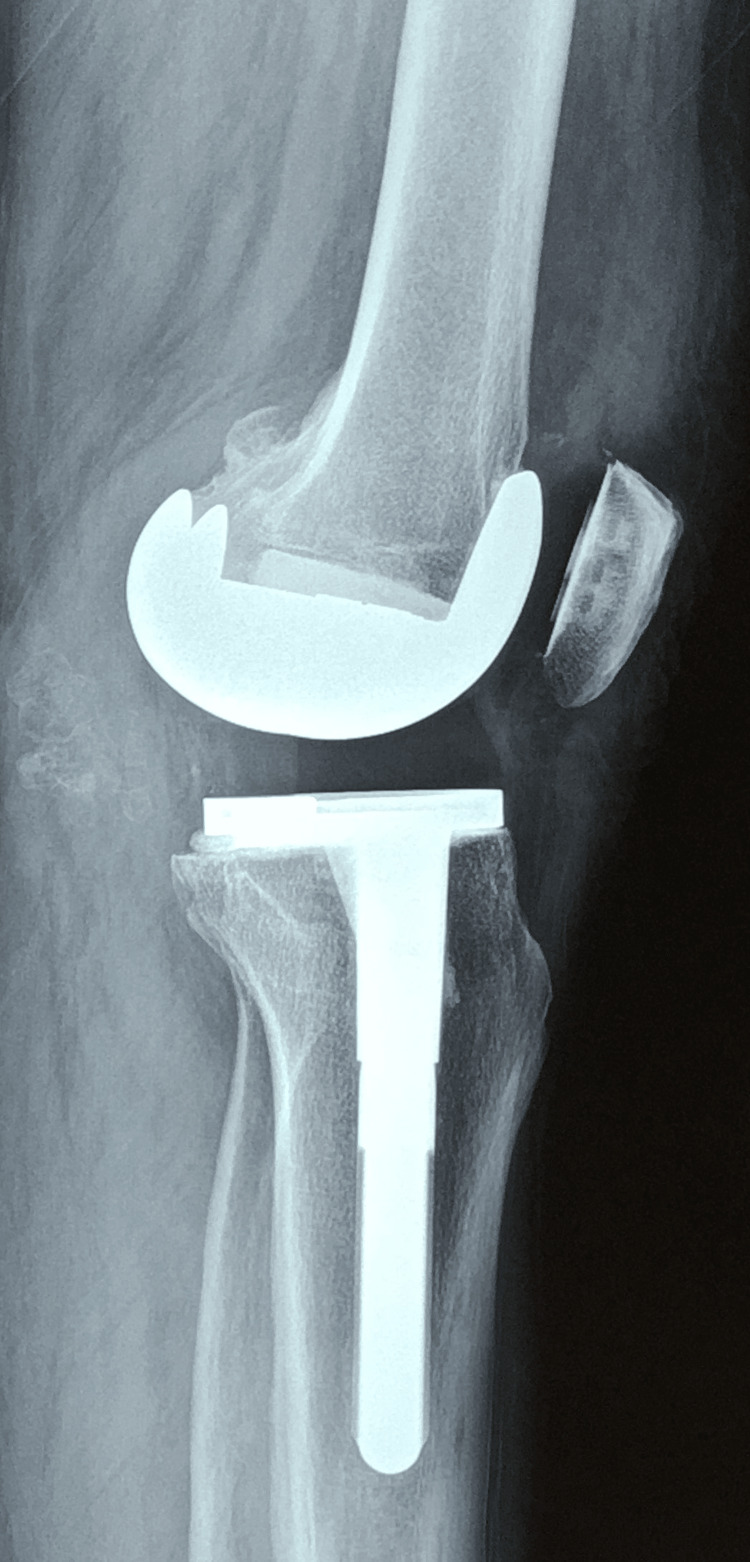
Two-year follow-up, lateral view X-ray For the X-ray, consent and permission were obtained from the patient.
